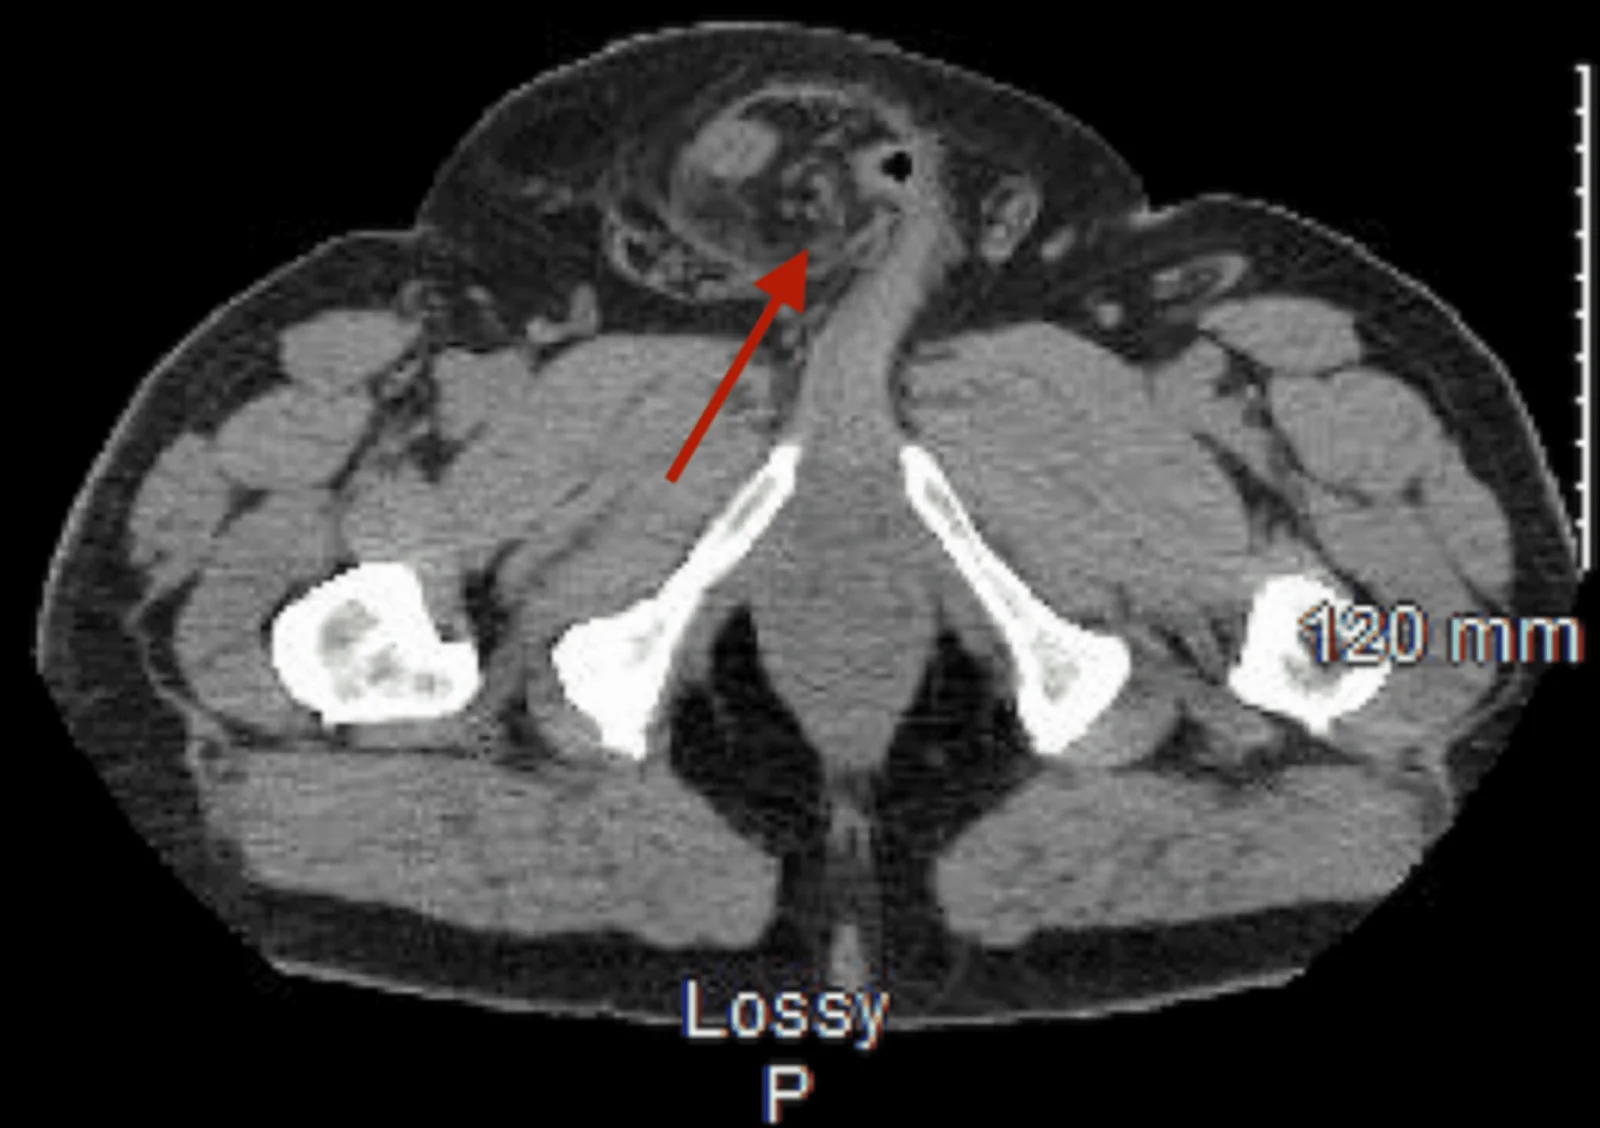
Inferior extension toward the right hemiscrotum. CT demonstrates inferior extension of the ventral hernia along the right lower abdominal wall toward the right hemiscrotum (red arrow).
